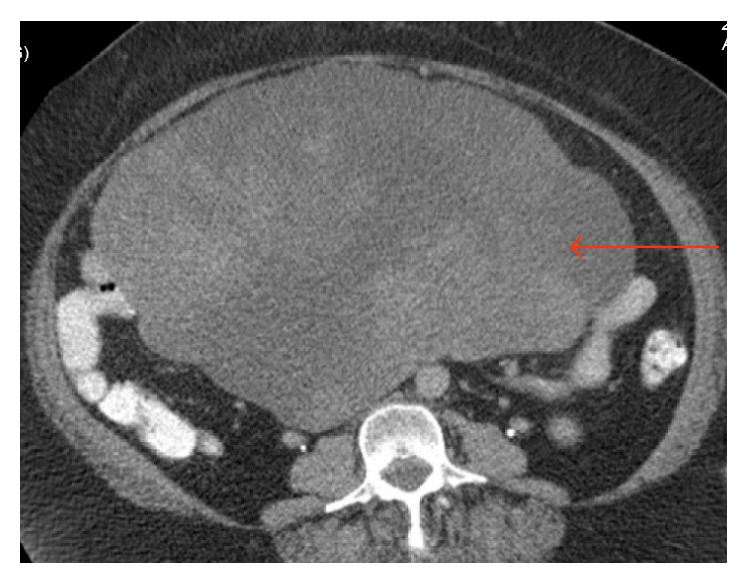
Abdomen CT scan with IV and PO contrast, axial view. Large multilobular mass (red arrow).
